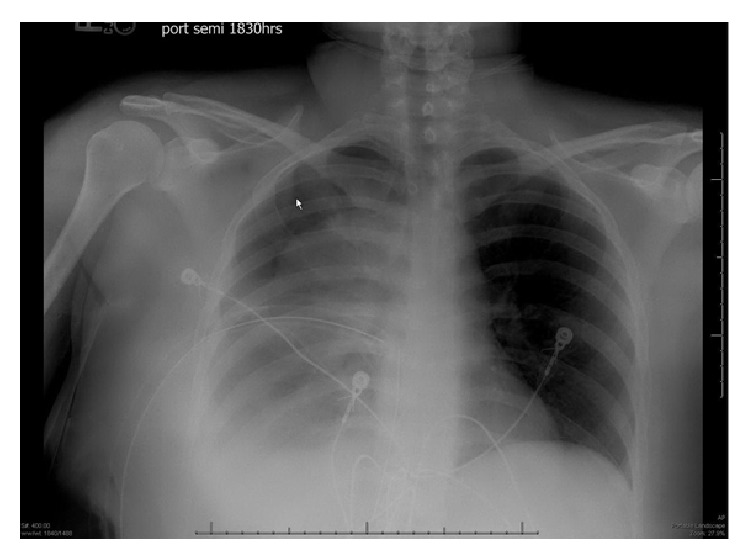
Chest X-ray obtained at trauma center showing persistent hemothorax despite chest tube placement at outside hospital.
